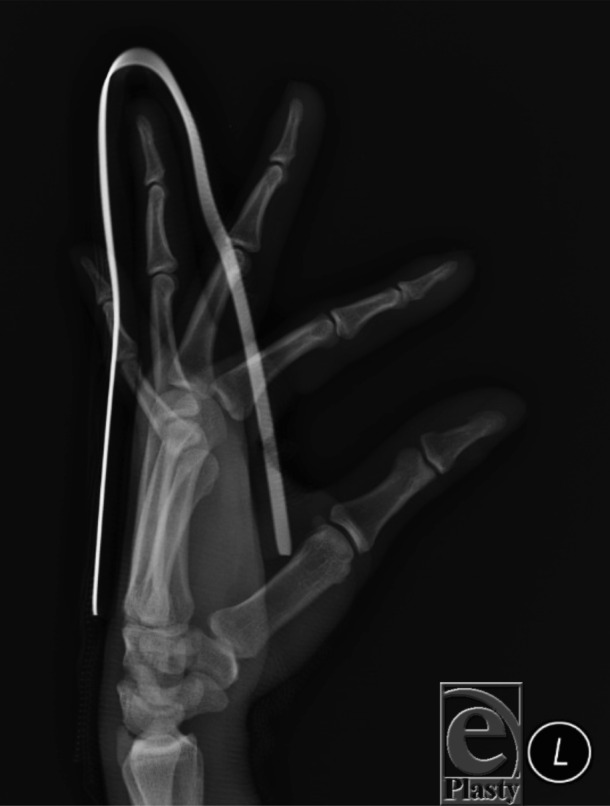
Postreduction radiograph and splinting of the affected finger in extension.
